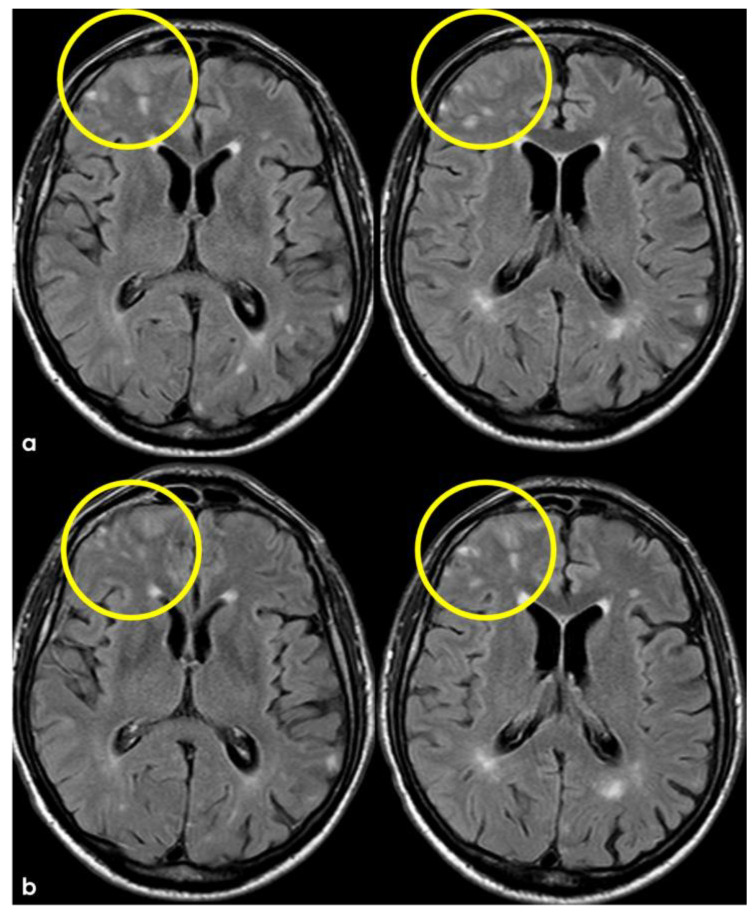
Brain MRI at 3 months from the previous one (Figure 3 and Figure 4), showing in axial FLAIR the persistence of spontaneous sulcal hyperintensity in the left anterior frontal lobe (panel (a), yellow circles) with contrast enhancement (panel (b), yellow circles). The remaining previously involved lobes (Figure 3) showed resolved sulcal involvement.
